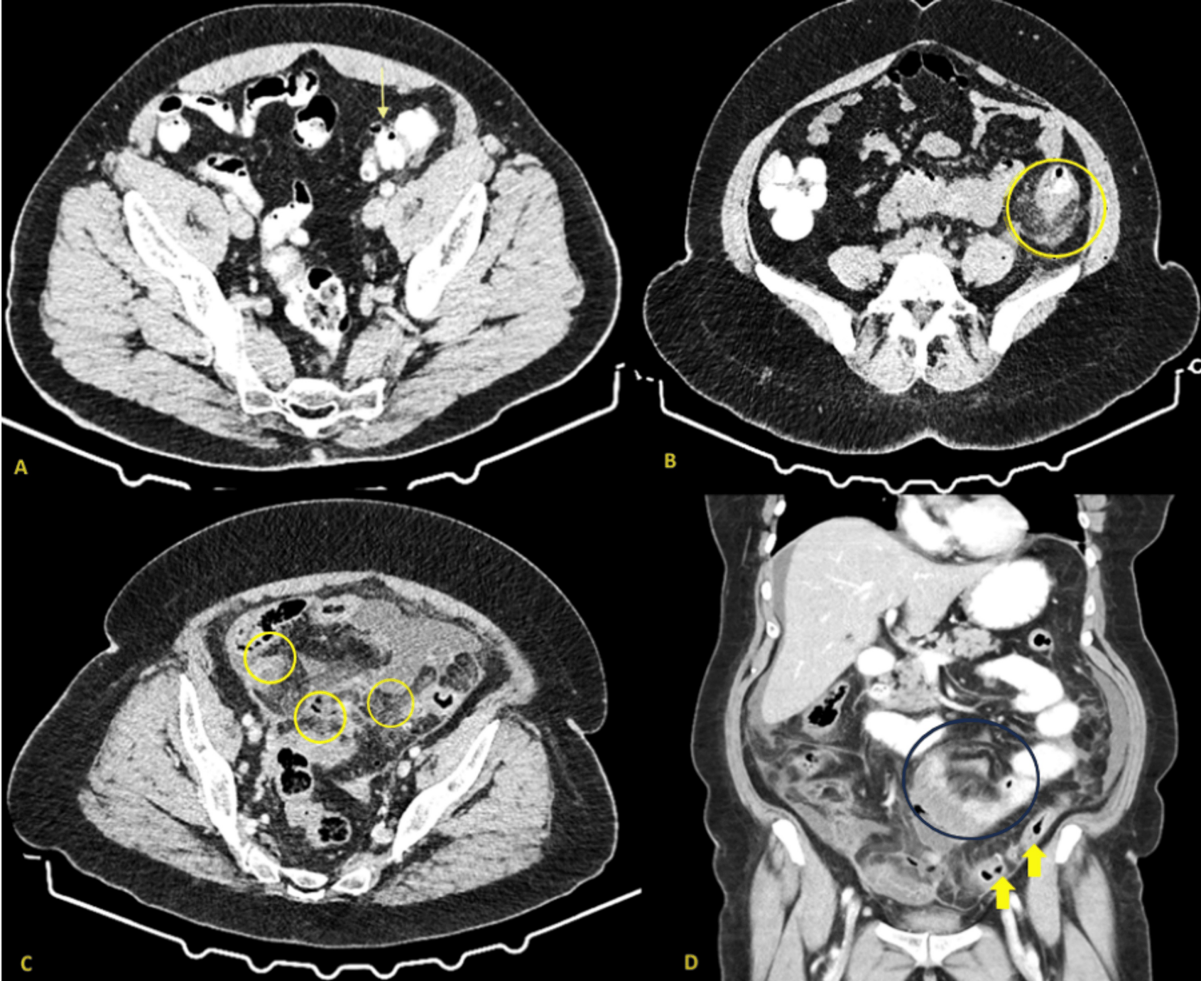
CT scans exhibiting different stages of diverticulitis (Hinchey 0-Hinchey 1b) (A) Hinchey 0 (uncomplicated diverticulitis): CT scan shows localized thickening of the sigmoid colon wall with diverticula (yellow arrow) without evidence of abscess, perforation, or pneumoperitoneum. (B) Hinchey 1a (pericolic phlegmon): CT scan demonstrates segmental colonic wall thickening of the descending colon with marked pericolic fat stranding and a small, ill-defined inflammatory phlegmon adjacent to the colon (yellow circle). There is no discrete abscess or distant fluid collection, characteristic of Hinchey 1a diverticulitis. (C (axial view), D (coronal view)) Hinchey 1b (pericolic abscess): CT scan reveals colonic wall thickening and pericolic fat stranding with well-defined, rim-enhancing pericolic abscesses measuring less than 5 cm in diameter (yellow eclipses and arrows). There was no evidence of distant abscess or generalized peritonitis, consistent with Hinchey 1b diverticulitis. Note the accumulation of small bowel dilated loops adjacent to the region of the inflammation causing paralytic ileus (black circle).
